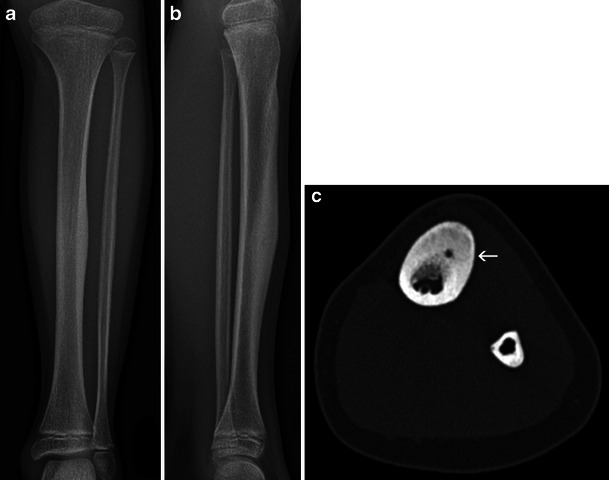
Osteoid osteoma of the antero-lateral tibial shaft: Frontal (a) and lateral (b) X-rays of the left tibia: osteosclerotic thickening of the antero-medial diaphyseal cortex. c Axial CT slice of the tibial shaft identifies a small lucent nidus (*arrow*) within the intense sclerotic cortical proliferation
